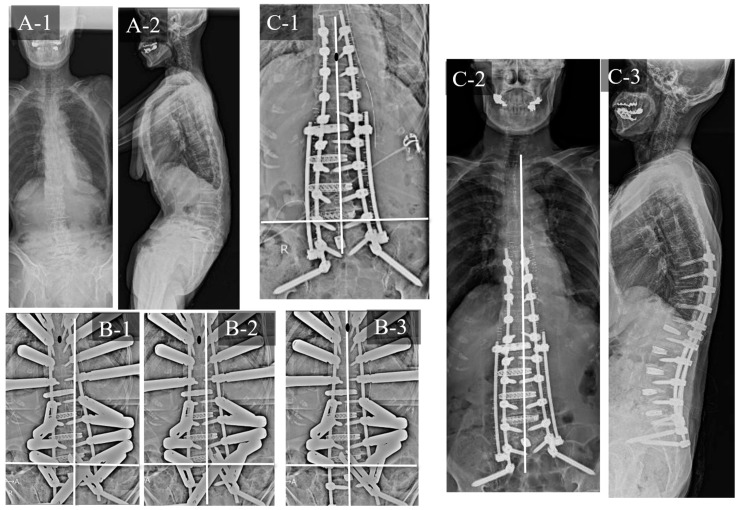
Case study 2 (RR and SD). (A-1) Preoperative standing whole spine radiograph (AP). (A-2) Preoperative standing lateral whole spine radiograph. (B-1) Intraoperative radiograph before RR. (B-2) Intraoperative radiograph after RR. (B-3) Intraoperative radiograph after SD. (C-1) Postoperative radiograph (AP). (C-2) Postoperative standing whole spine radiograph (AP). (C-3) Postoperative standing lateral whole spine radiograph. RR: rod rotation, SD: sacral alar-iliac screw distraction technique.
